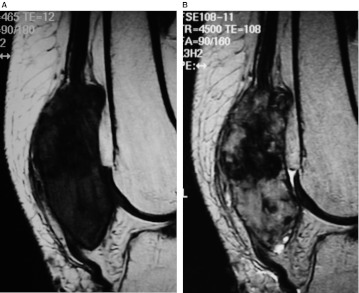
Magnetic resonance imagines showing an intraosseous lesion extending into the anterior soft tissue. A: On T1-weighted images, the lesion showed low signal intensity. B: On T2-weighted images, the proximal part of the lesion showed low signal intensity with partial high-signal areas, and the distal part showed high signal intensity with focal low-signal areas.
